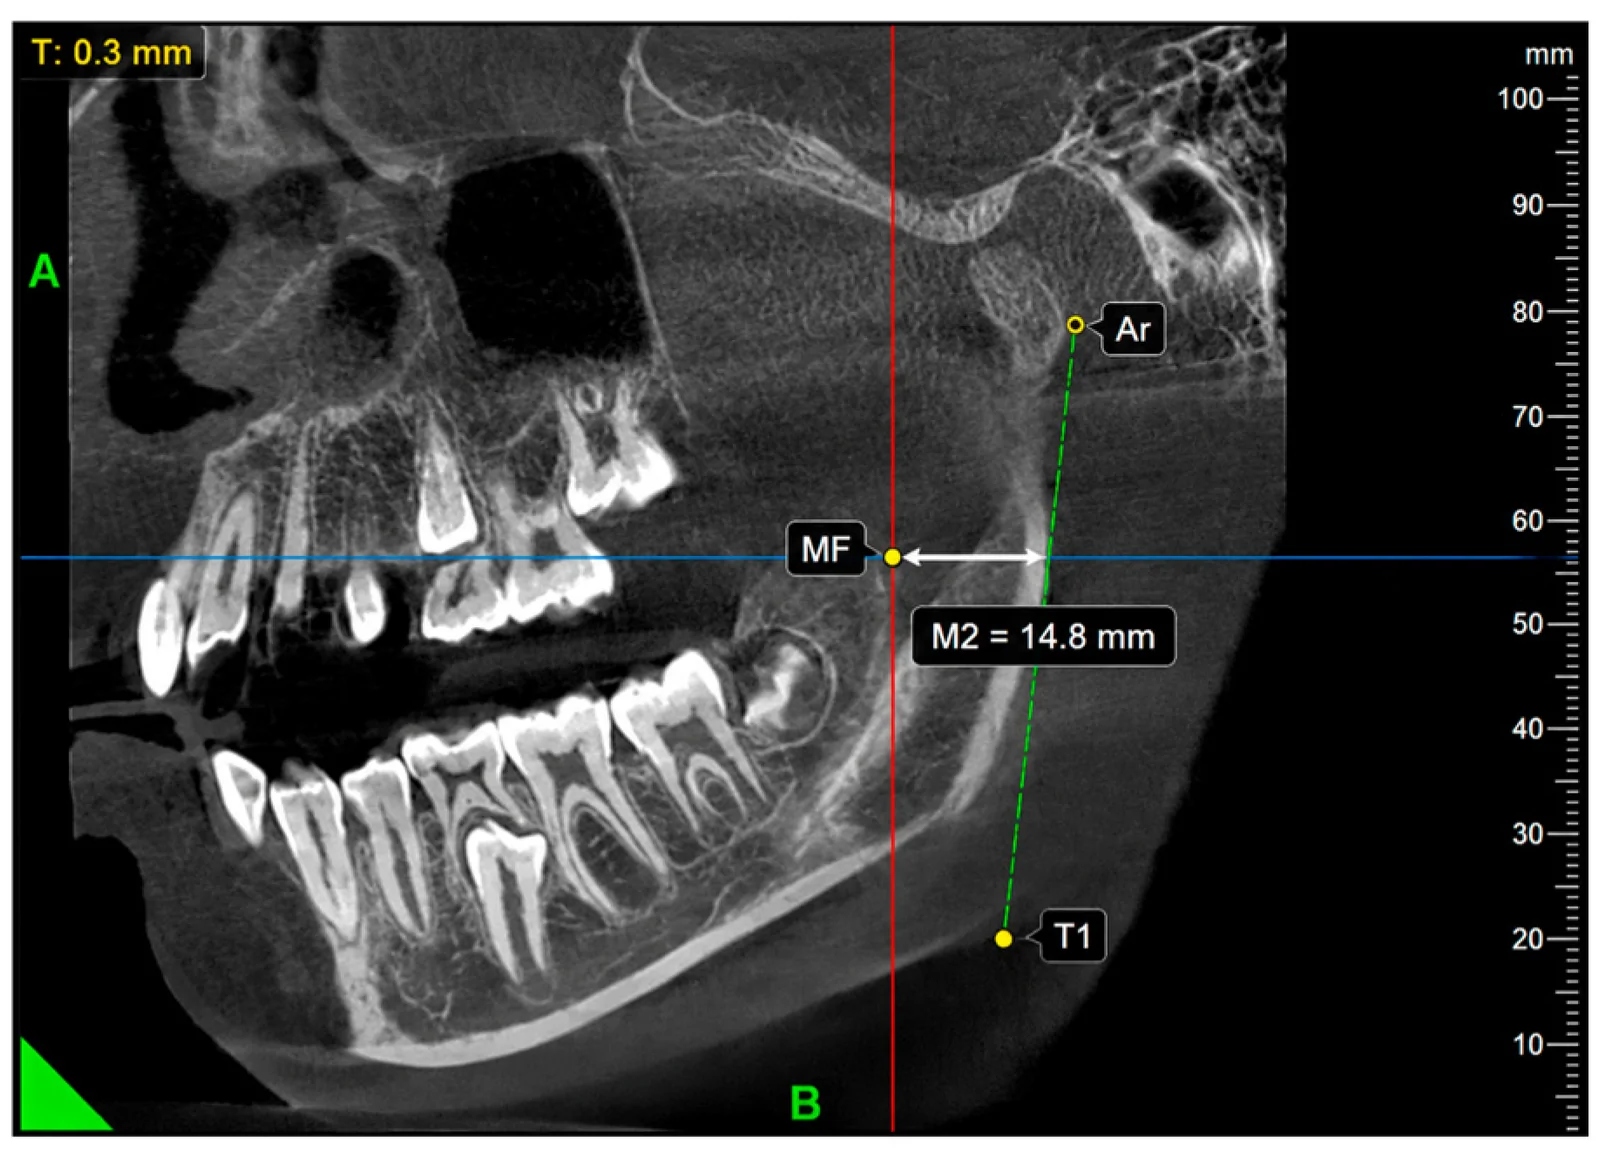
Sagittal CBCT image showing the M2 measurement (mm), defined as the shortest perpendicular distance from the fixed superior mandibular foramen reference point (MF) to the posterior ramal reference line connecting articulare (Ar) and the posterior tangent point (T1).
